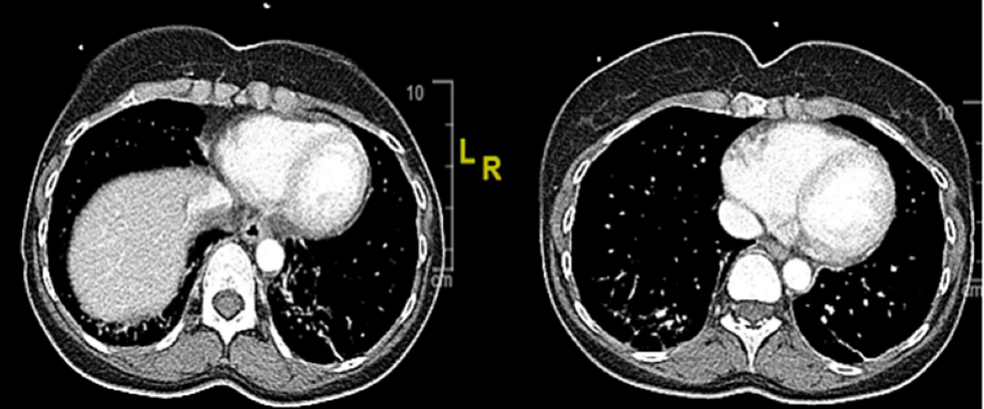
Axial chest computed tomography showing patchy opacities posterior within both lower lobes.
